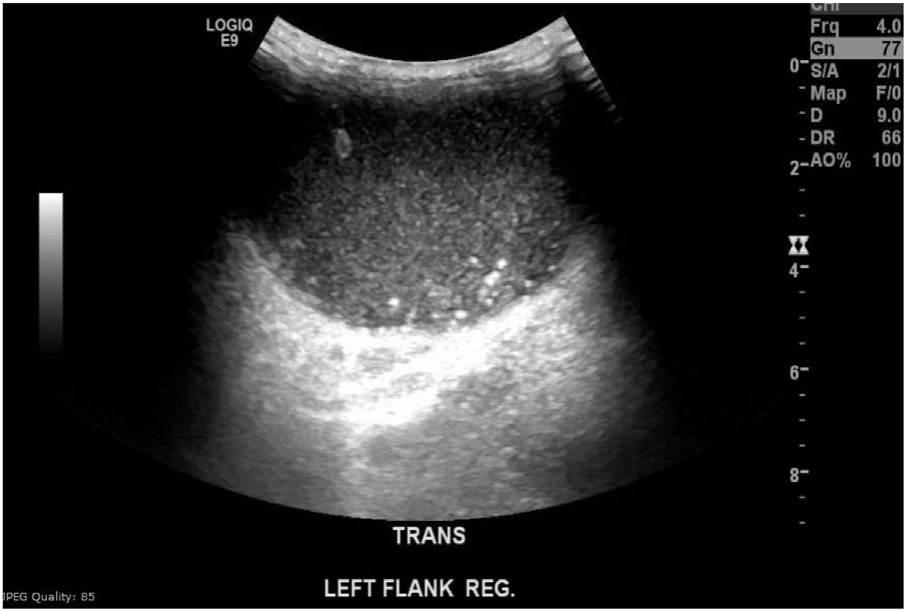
Ultrasound examination of the lesion in the lower chest wall showed well defined hypoechoic area, with varying degrees of internal heterogeneity.
